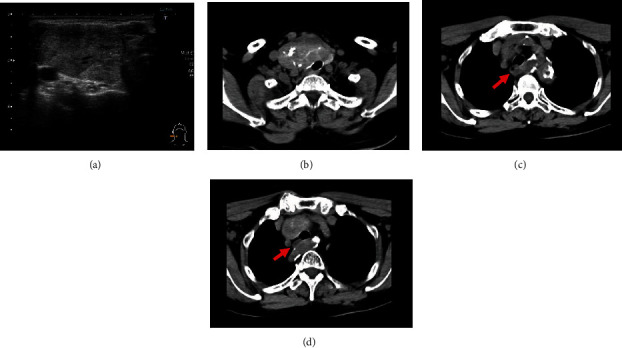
Preoperative imaging. (a) Ultrasonography showing a mass in the right thyroid lobe. (b) Computed tomography image showing the thyroid tumor with calcification compressing the cervical trachea. (c, d) The right aberrant subclavian artery (arrow) running behind the esophagus (^∗^).
